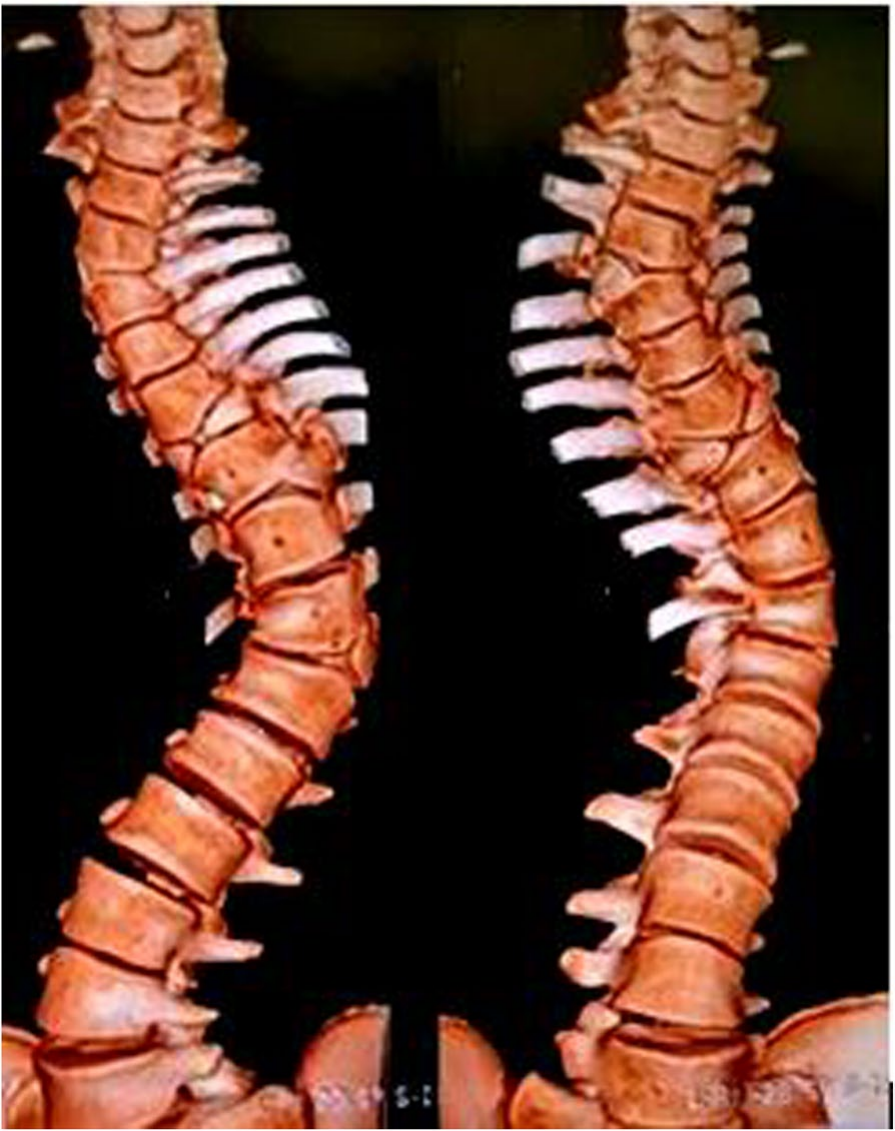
Pre-operative 3D reconstruction of CT scan showing the curve and the congenital disorder of segmentation in the form of hemivertebrae, butterfly vertebra. 3-dimensional anatomy of the curve is better appreciated
